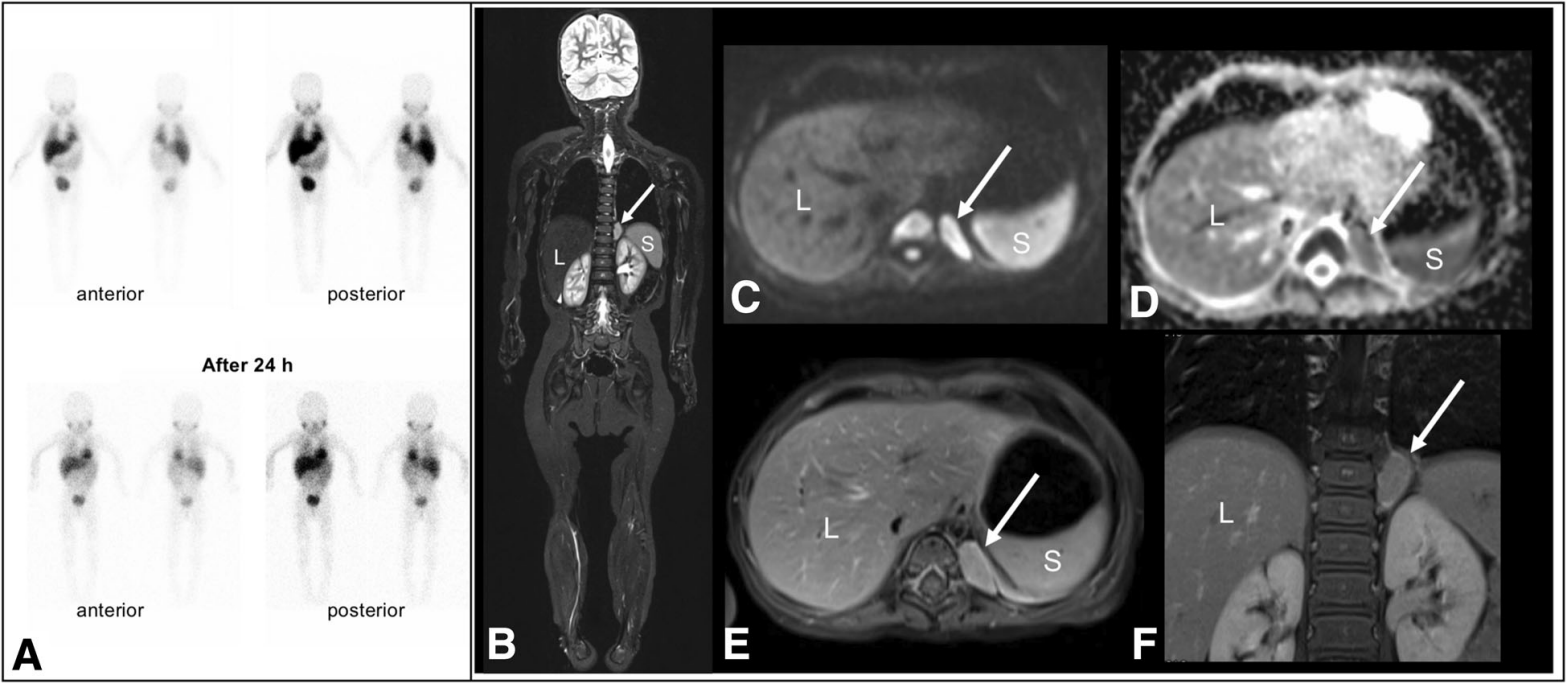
Case 1: 17 months old girl presenting with opsoclonus-myoclonus syndrome. Whole-body I-123-mIBG scintigraphy with 60 mBq I-123-mIBG did not reveal any pathological tracer uptake (a). WB-MRI revealed a solid left-sided paravertebral mass extending to the correlated neuroforamina (*white arrow*) at the level of thoracic vertebrae T 9/10 with signal hyperintensity in T2 weighted Turbo-Inversion Recovery-Magnitude (TIRM) sequences (b). In diffusion weighted imaging, correlated restricted diffusion could be detected with low ADC (c and d) and strong enhancement with hyperintensity could be detected in the T1 weighted sequence after the administration of 2 ml gadolinium compound (e and f). *L = liver, S = spleen*
